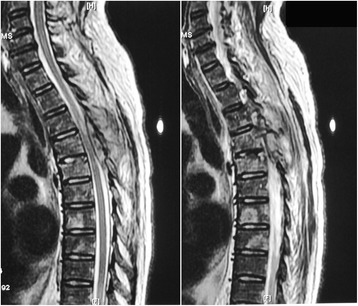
Bone destructions and compressed spine: These two sagittal views of spinal MRI T2-weighted sequence revealed multiple bone destructions of thoracic vertebrae, with spine compressed at T4 and T5 level
